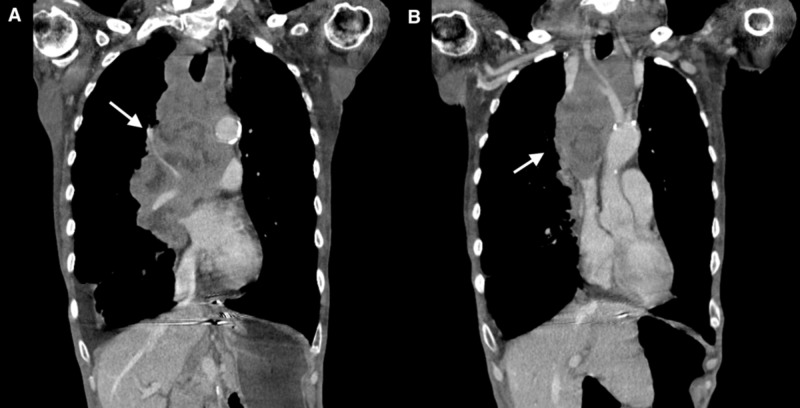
CT chest with contrast revealing large mediastinal mass (A) involving the entire mediastinum and the right hilum and causing (B) narrowing of the superior vena cava.
